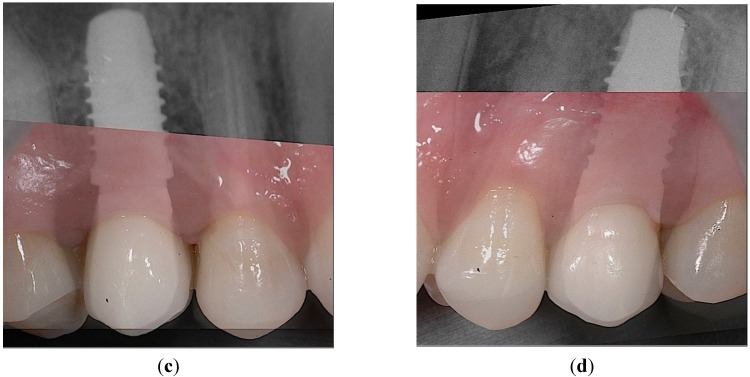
Intraoral views at one-year follow-up. (a) The optimal gingival margin was formed beneath the permanent crowns, both in the case of PFS, (b) as well as the StE implants. Superimposed clinical and X-ray pictures: (c) Position 15, PFS; (d) Position 25, StE.
